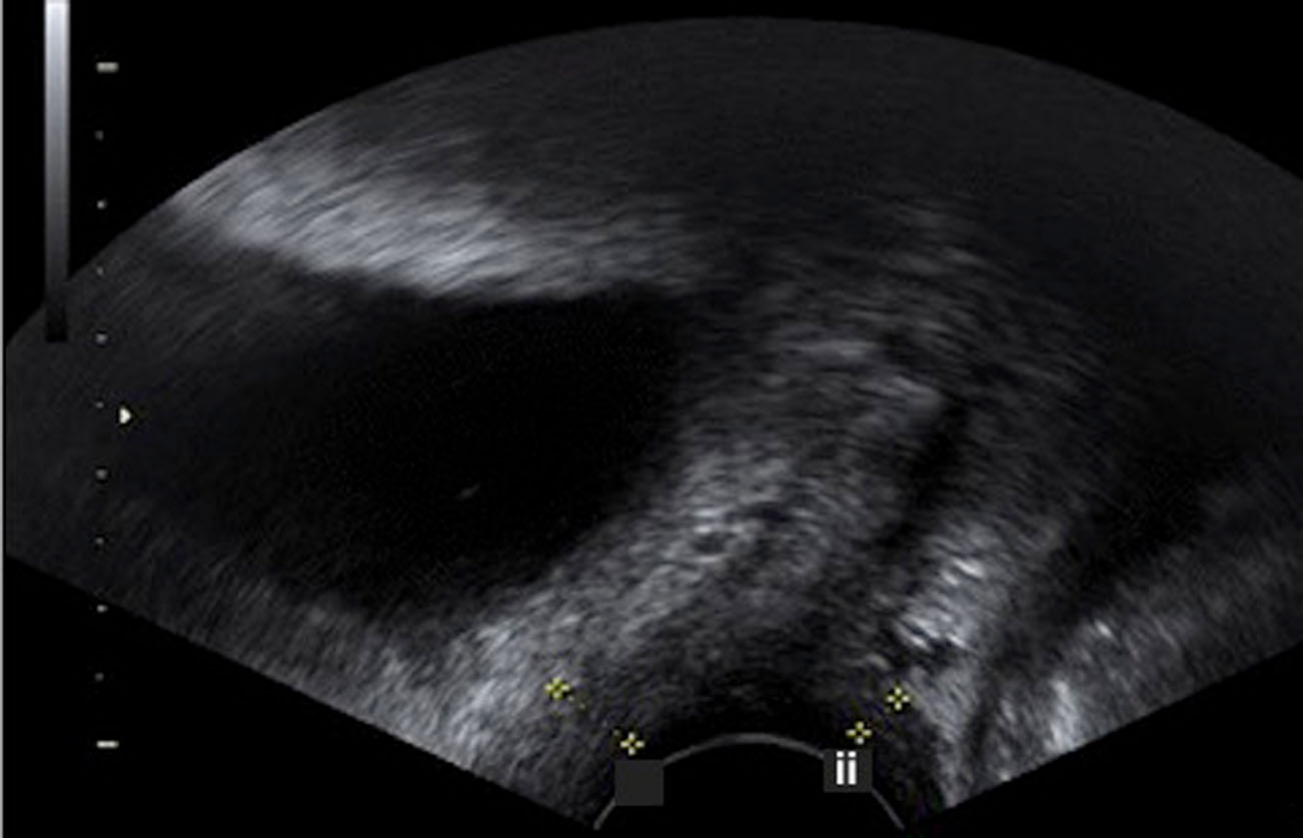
Ultrasound image showing measurement of vaginal wall thickness at the level of the bladder neck *i* and the anorectal junction *ii*
